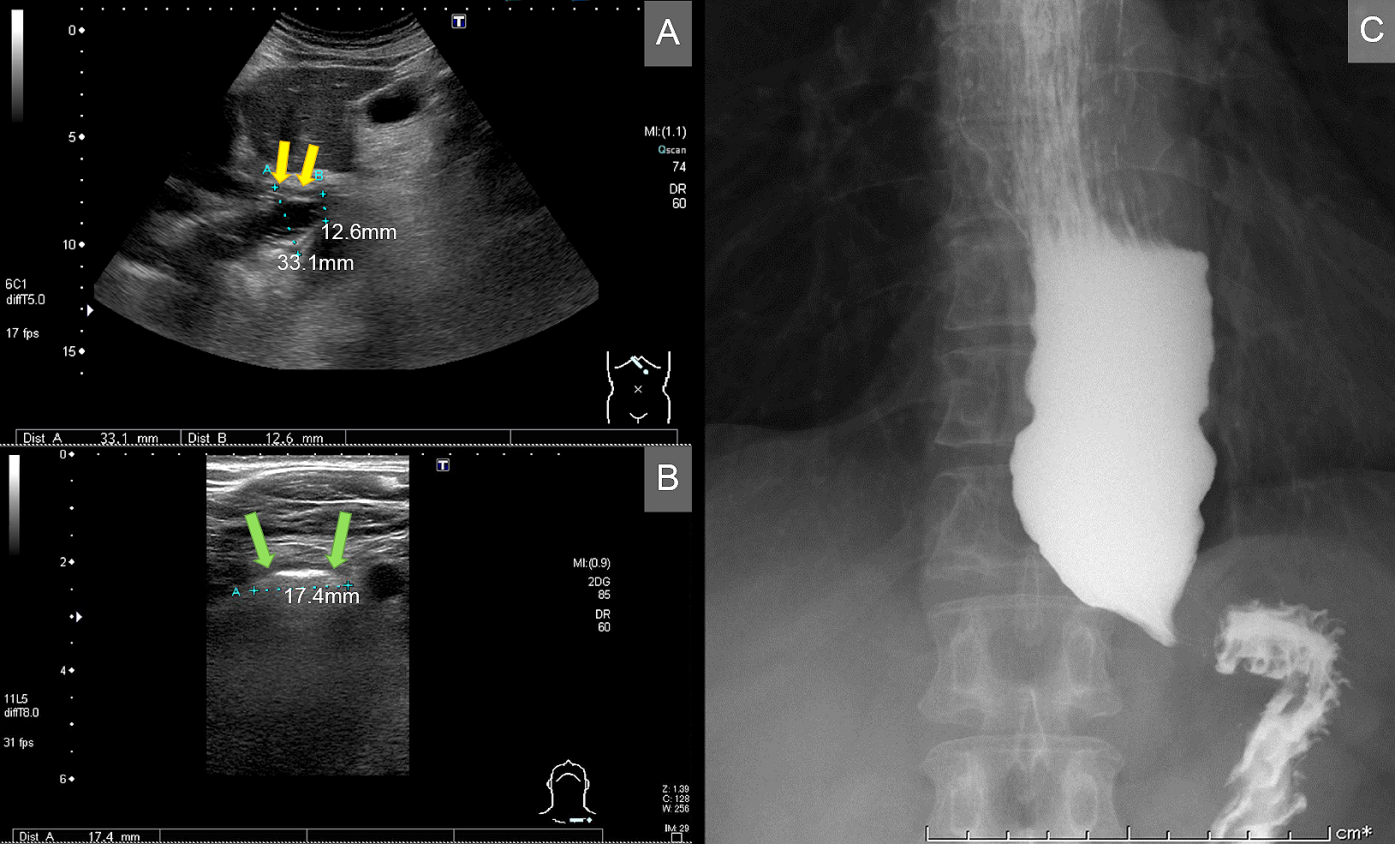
A transabdominal and neck ultrasonogram showing a dilated esophagus. (A) Significant dilatation and narrowing of the distal segment of the esophagus, with regular hypoechoic wall thickening (yellow arrow). (B) A neck ultrasonogram showing the dilated esophagus (green arrow). (C) A barium esophagogram showing the dilated esophagus, consistent with achalasia.
